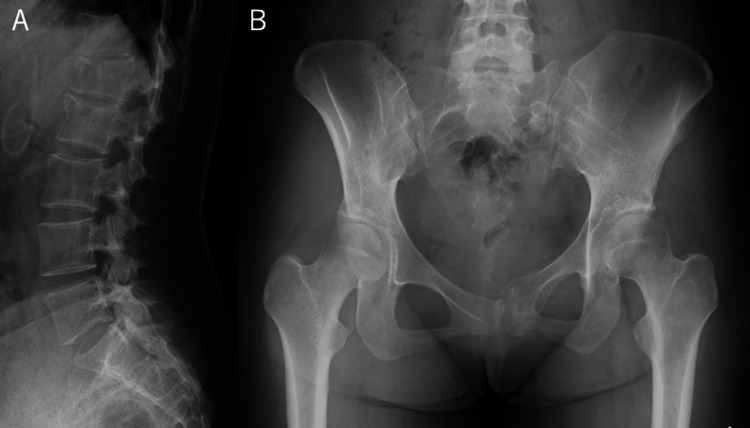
Imaging findings after implant removal. (A) Lateral X-ray view after implant removal. (B) Frontal X-ray view after implant removal.
